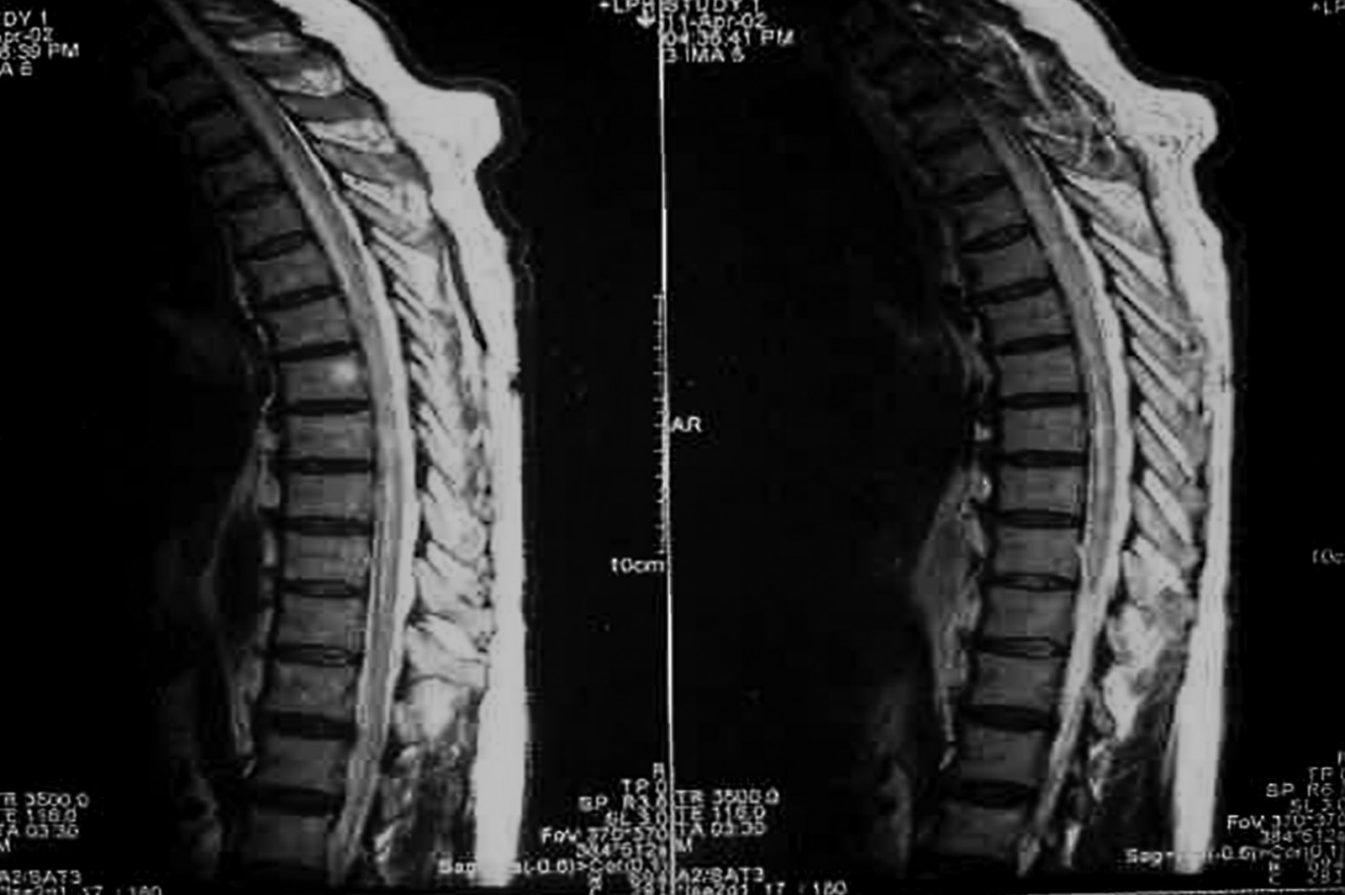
Magnetic resonance image (sagittal plane, T2 sequence): Extensive enhanced signal at dorsal spinal cord in a patient presenting acute myelitis caused by dengue infection.
